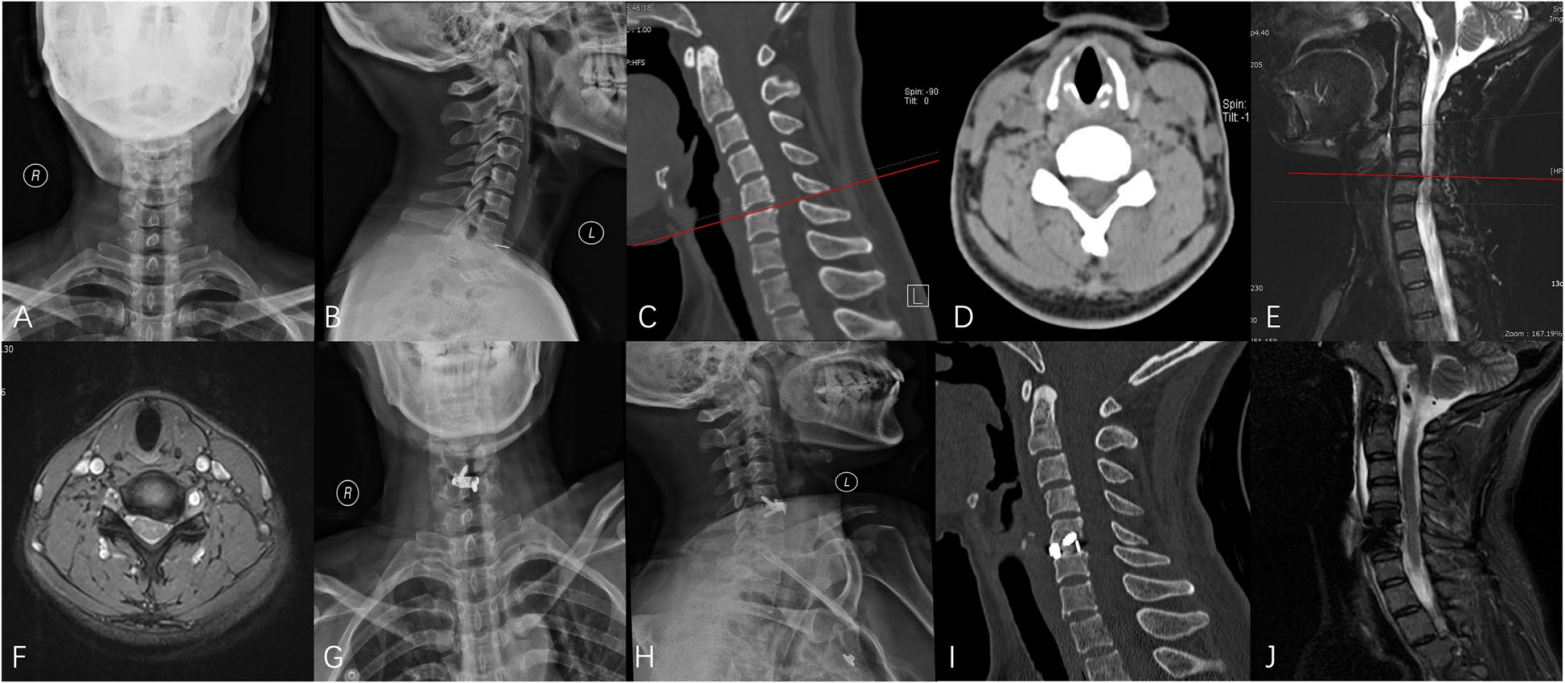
A, B The preoperative A-P X-Ray showed the height of C5/6 decreased, C The preoperative CT sagittal showed cervical lordosis, the height of C5/6 decreased with posterior edge osteophyte hyperplasia, D The preoperative CT coronal view showed disc herniation of C5/6, E, F The preoperative MRI showed disc herniation of C5/6 (left), G, H The postoperative A-P X-ray showed a good cervical physiological curvature, I The postoperative CT showed a perfect position of the Zero-P implant, J The postoperative MRI showed the spinal cord obtained a full decompression with smooth cerebrospinal fluid circulation
